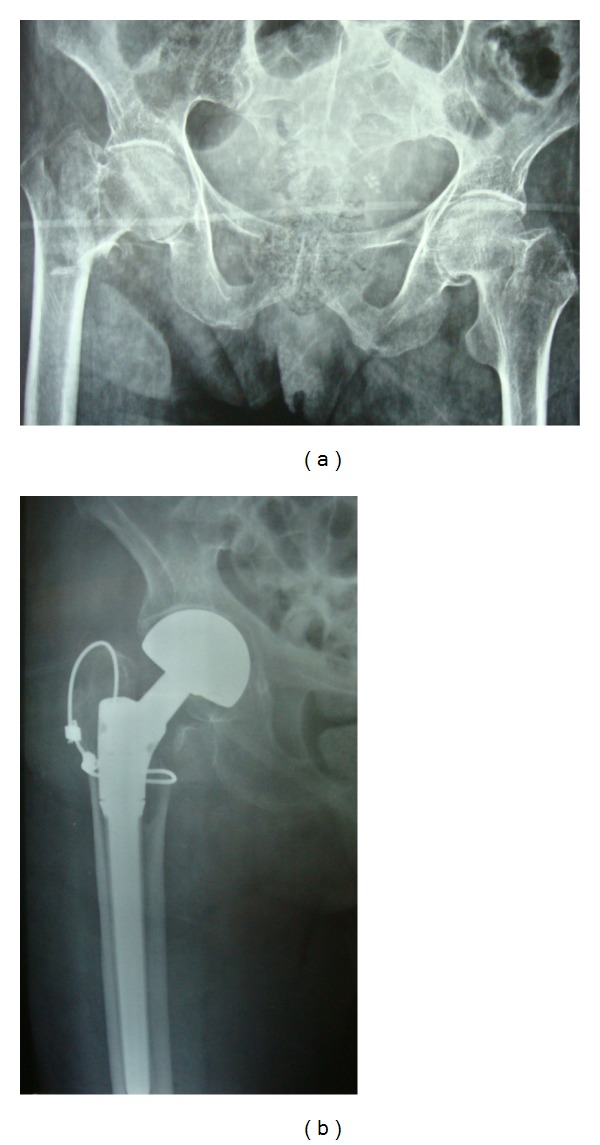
Radiographic images of a hip fracture. (a) Before surgery. (b) At 6 months after fixation with a cable.
